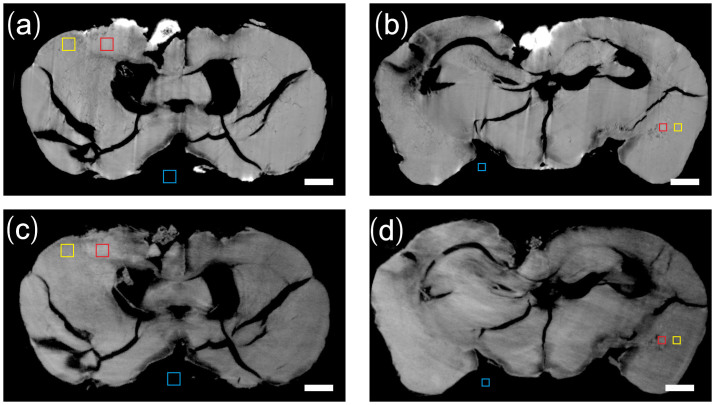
Three regions selected in IPC-CT image (a, b) and GPC-CT iamge (c, d) for CNR analysis. (1) red square: tumor (2) yellow square: surrounding region of the tumor (3) blue square: background region. The areas of the square regions in (a), (c) are 200 × 200 μm^2^ while 120 × 120 μm^2^ in (b), (d). Scale bar: 500 μm.
